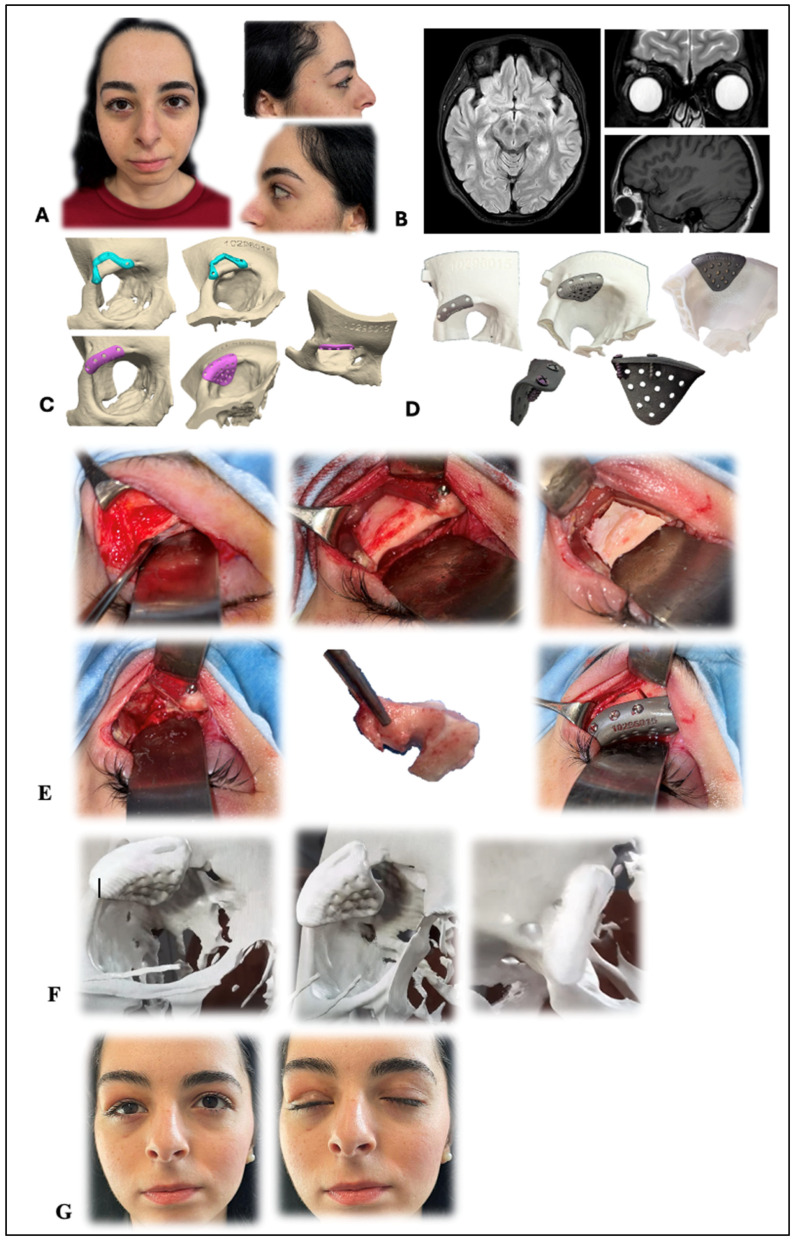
(A) Preoperative photos showing the asymmetry caused by the right-side proptosis. (B) MRI showing an extraconal dermoid cyst in the right orbit with mild mass effect and bone remodelling. (C) Virtual surgical planning with orbit and lesion segmentation for cutting guide and PSI fabrication. (D) Titanium cutting guide, PSI, and 3D models—was produced in-house. (E) Sequence of images read from right to left and top to bottom: (1) Right upper blepharoplasty approach with subperiosteal exposure. (2) Positioning of titanium cutting guide. (3) Planned osteotomy with piezoelectric device. (4) En bloc resection of extraconal lesion. (5) Resected dermoid cyst. (6) Placement of PSI over repositioned bone flap. (F) Intraoperative CT confirming resection, defect adequacy, and PSI placement/fit. (G) Clinical images of the patient at 2-month postoperative follow-up.
